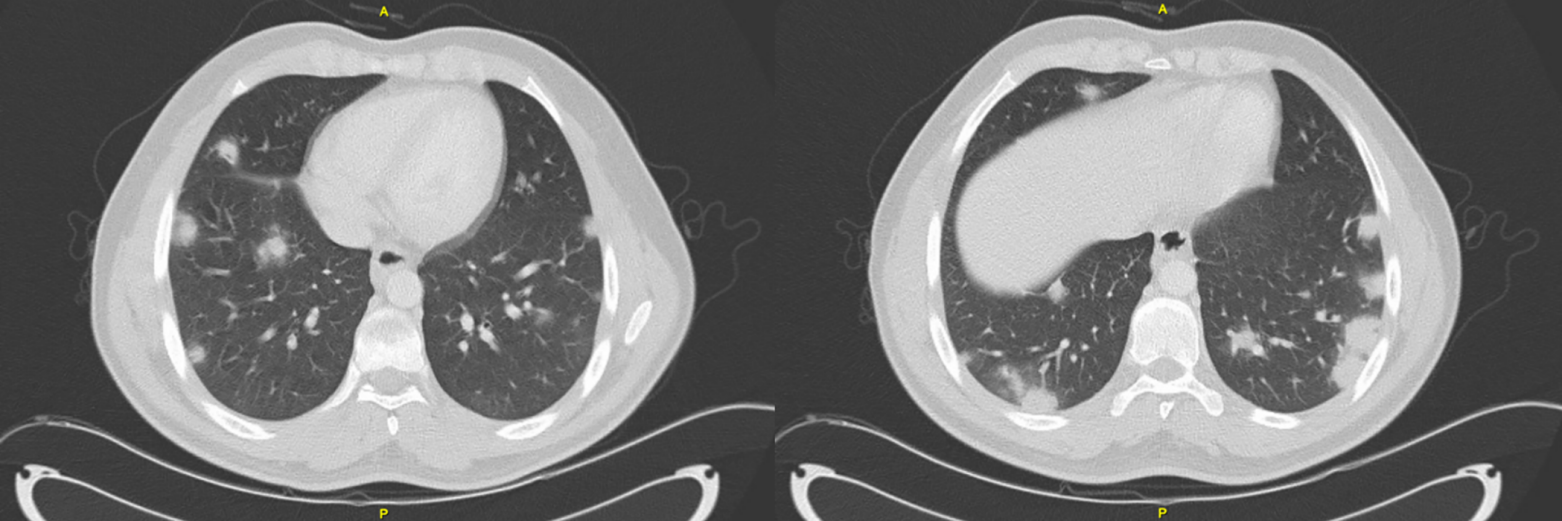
Contrast‐enhanced CT chest showing multiple poorly marginated, irregular contoured, enhancing nodules throughout both lungs.
